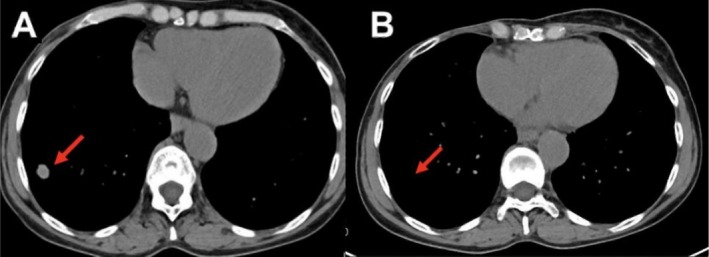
(A) Axial reconstruction of computed tomography scan showing a 15 mm nodule in the right lower lung lobe (March 2019). (B) The same level axial reconstruction in 2020 showed a complete disease response.
